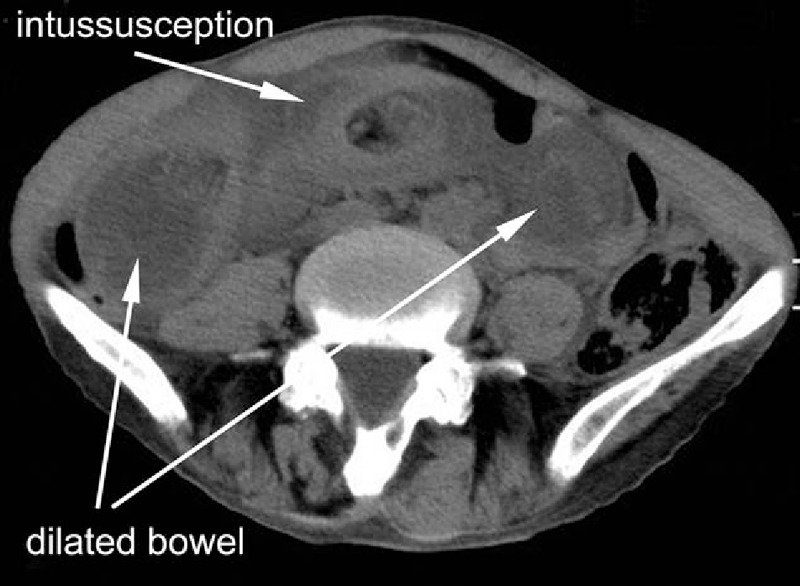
Computed tomography showed a typical image of intussusception, together with dilated jejunal loops.
